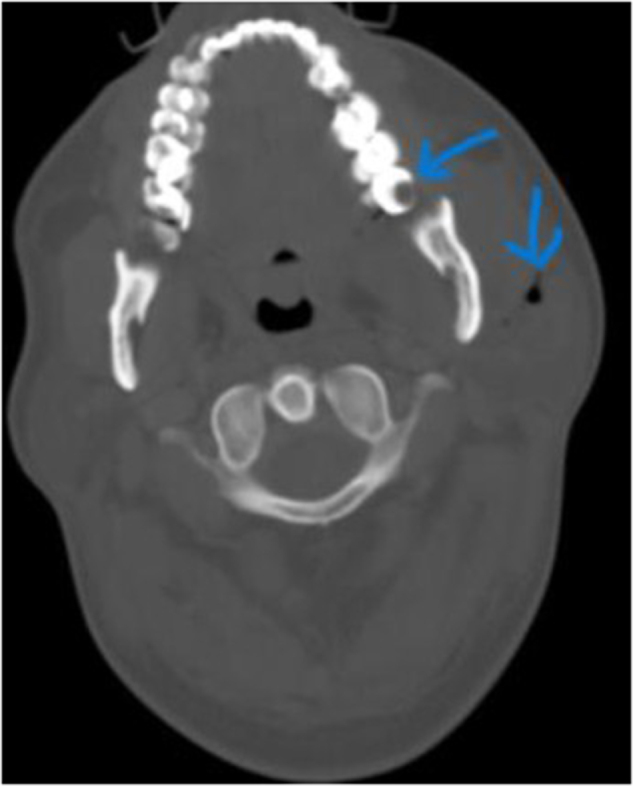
Axial bone CT scan image showing a last molar dental cavitation and periodontal abscess.
